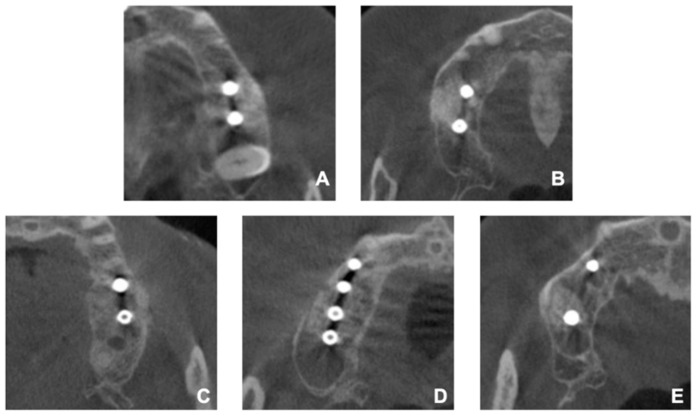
CBCT images of the patients after the operation. (A) Patient 1, (B) Patient 2, (C) Patient 3, (D) Patient 4, (E) Patient 5.
